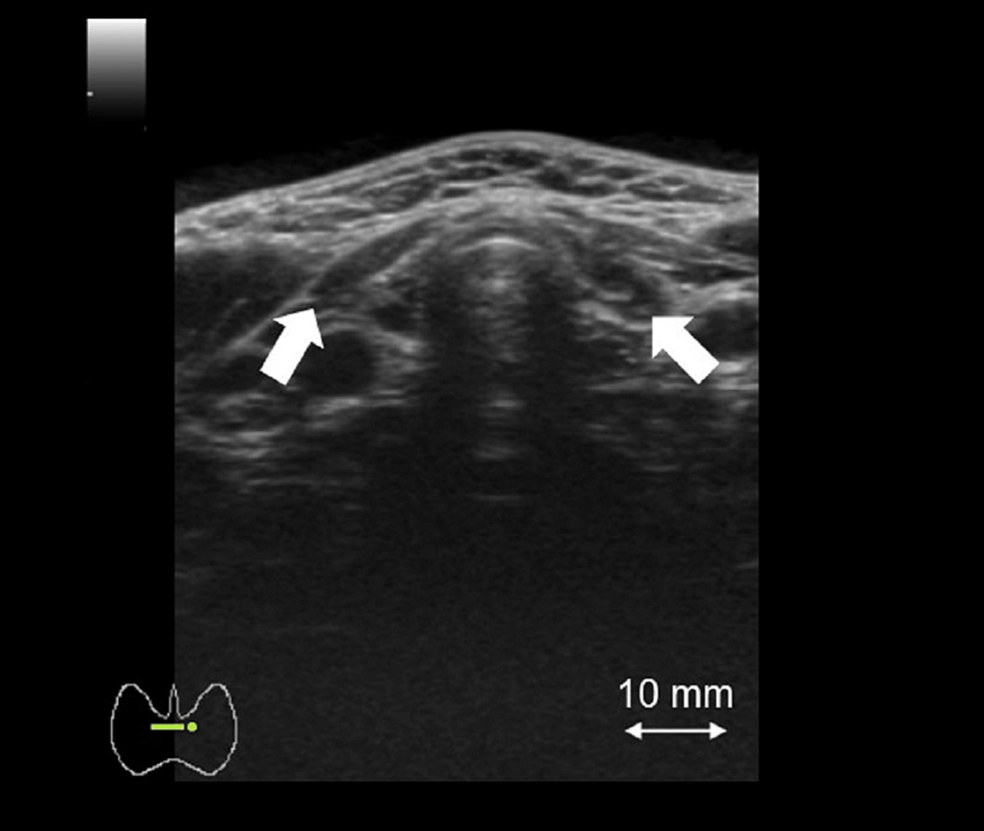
Thyroid ultrasonography. Ultrasonographic findings of the thyroid gland demonstrating the remarkable shrinkage and irregular surface and heterogeneous low-level internal echoes without high Doppler blood flow at nine years after initial presentation (white arrows, right lobe 44×7.4×7.1 mm, left lobe 46×5.6×6.6 mm, isthmus thickness 1.4 mm).
